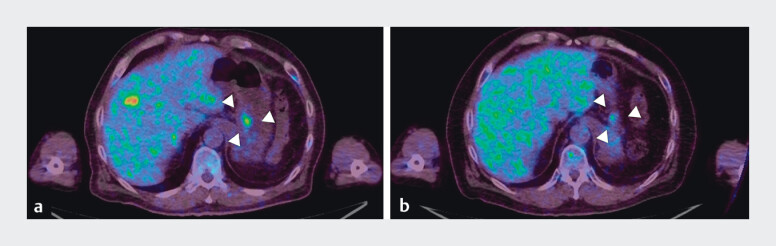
Positron emission tomography–computed tomography (PET–CT) (delayed-phase) demonstrating
